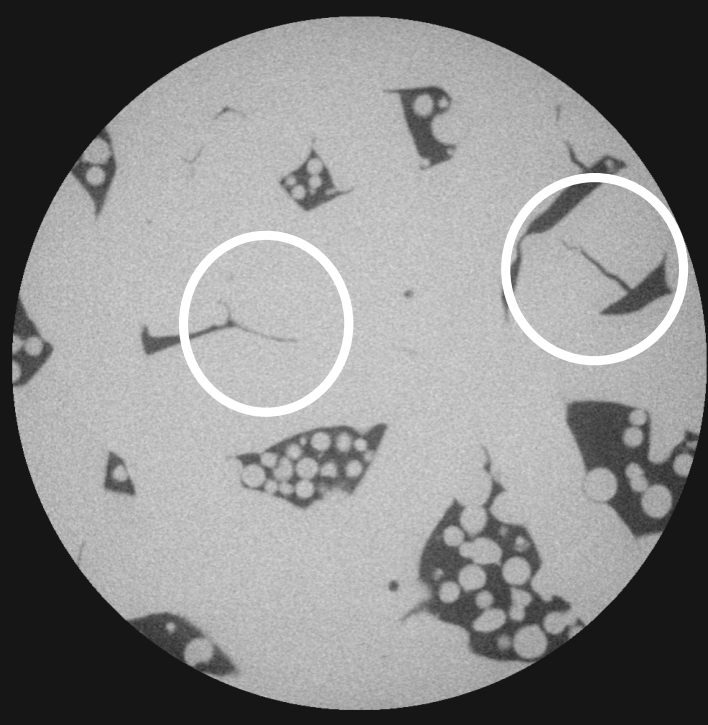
Sample 1–5D, X-ray CT of the interior of a 5 mm diameter cylinder cut from the original sample. The 5 mm cylinder had the same height as the original sample, but the image stack only extends part way through the sample. The pixel size in this image is 0.87 µm. Table 7 gives more details about the images taken from this sample. The white circles mark apparent cracks.
